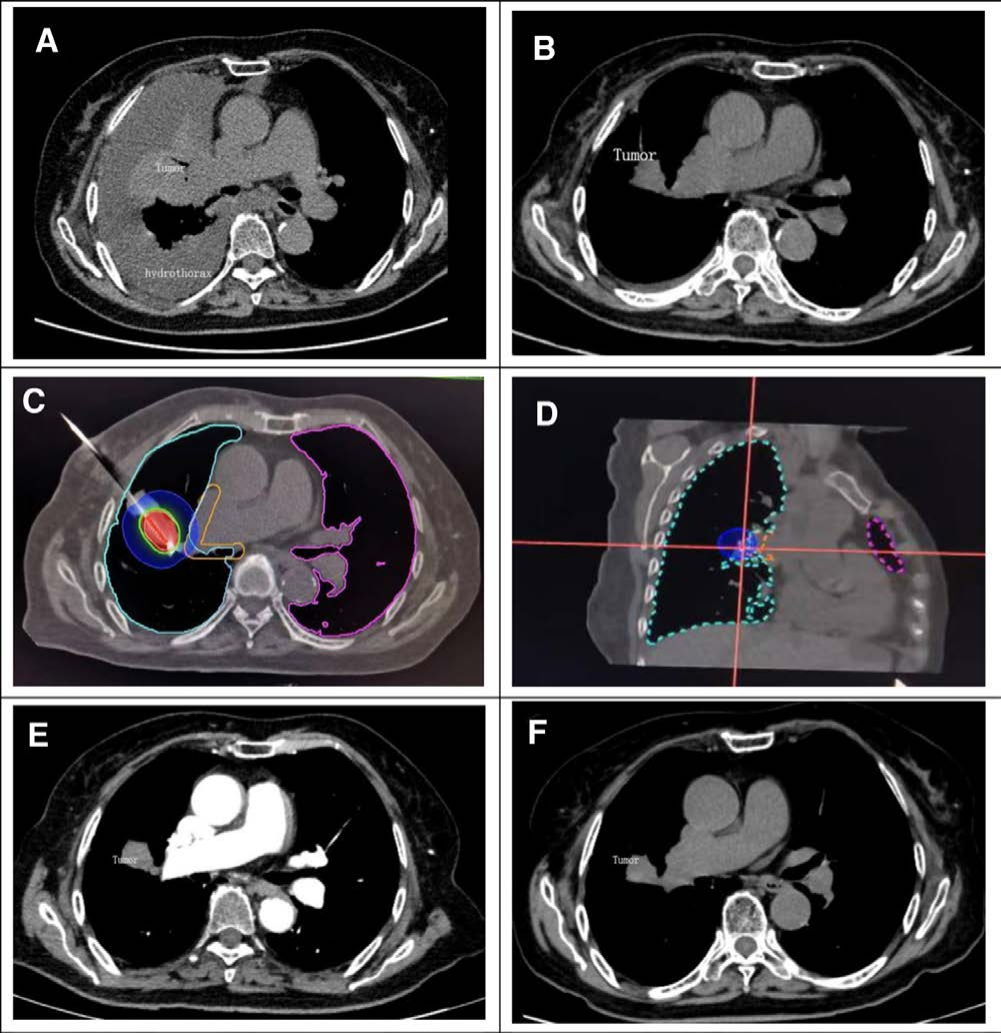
Changes observed in the patient before and after receiving HDR ^192^Ir brachytherapy. (A): CT at the time of admission revealed a 4.8 × 4.2 cm right thoracic tumor lesion with a large amount of pleural effusion; (B): Only a small amount of pleural effusion was left before HDR ^192^Ir brachytherapy; (C and D): A single dose of 27 Gy to the target lesion; (E): After 2 weeks post-treatment, the size of the lesion was reduced to 3.0 × 2.3 cm; (F): After 18 months of treatment, the lesion showed no progression. Gy = gray, HDR = high-dose-rate.
